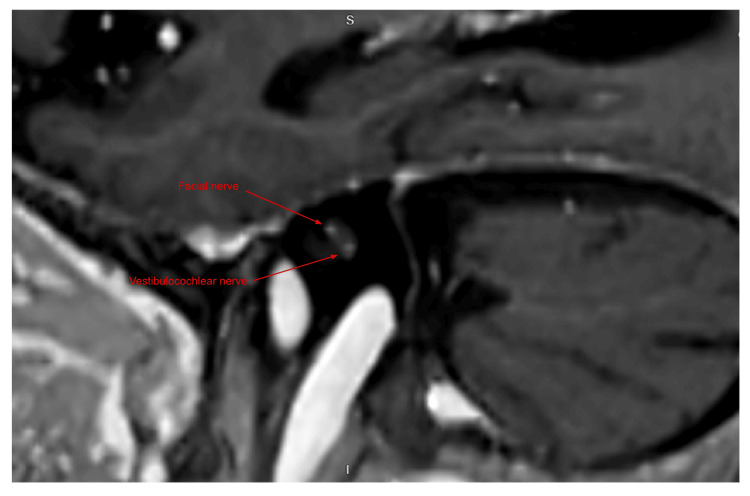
Sagittal T1-weighted post-contrast MRI of the internal auditory canal. The red arrows identify enhancement of the facial nerve (located anterior-superiorly) and the vestibulocochlear nerve (specifically the anterior-inferior cochlear branch), consistent with Ramsay Hunt syndrome.
